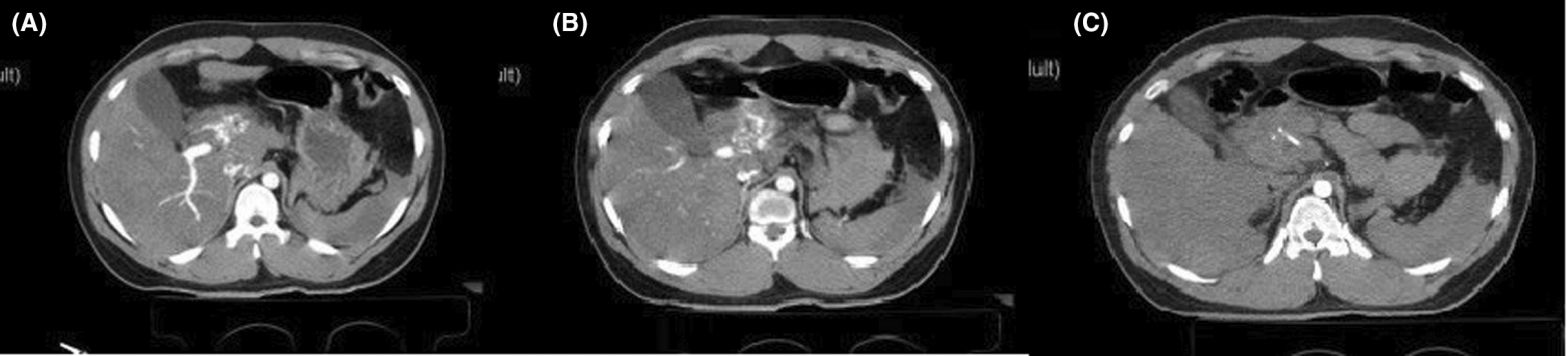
Axial contrast‐enhanced computed tomography images acquired showing contrast material extravasation in the porta hepatis area. (A, B) The dual‐phase (portal‐venous and arterial phase) image demonstrates extravasation of contrast material in the porta hepatis area and moderate free fluid in the abdominal cavity. (C) The arterial phase reveals evidence of hematoma formation in the porta hepatis area around the proper hepatic artery. Free fluid accumulated in spaces adjacent to the spleen is observed on the mentioned plains of the CT scan.
